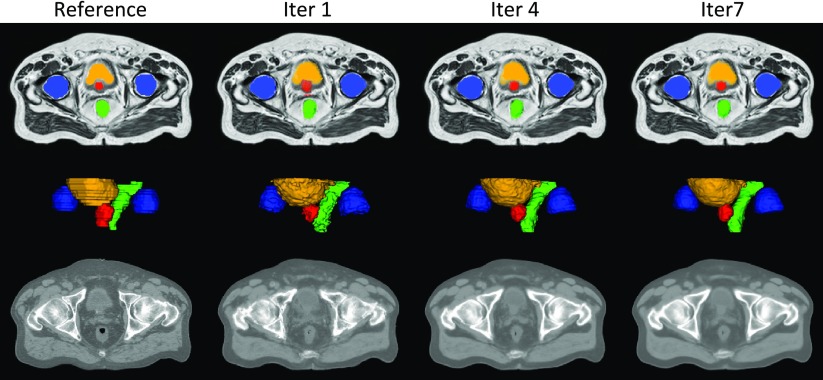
The probabilistic (top) and categorical (middle) segmentations of the prostate (red), bladder (orange), rectum (green) and femur heads (blue), and the pseudo CTs (bottom) were obtained from the T1-weighted and T2-weighted MR images of the patient. Note how the smoothness of the segmentations and sharpness of the pseudo CTs increase with the number of iterations.
